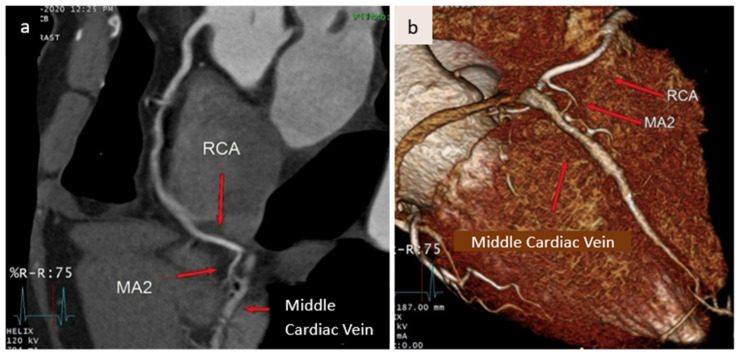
Small arteriovenous fistula between an acute marginal branch (MA2) of the right coronary artery (RCA) and the middle cardiac vein in a 58-year-old woman in computed tomography curved planar reconstruction (a) and volume-rendering (b) images.
